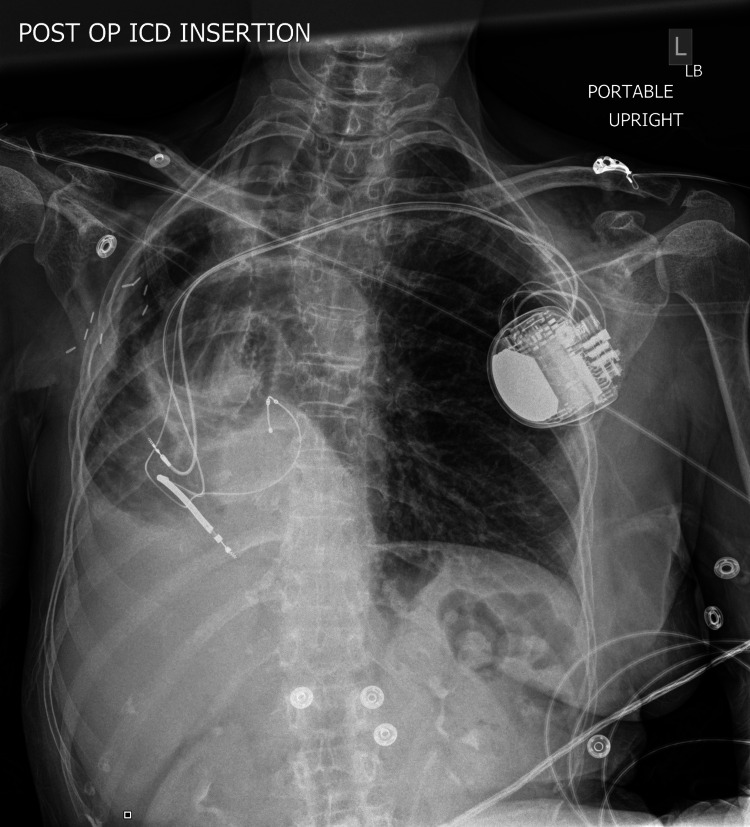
Chest X-ray (posteroanterior (PA) view) Post-ICD implantation leads and pseudo-dextrocardia are visible in the image. ICD: Implantable cardioverter-defibrillators.
